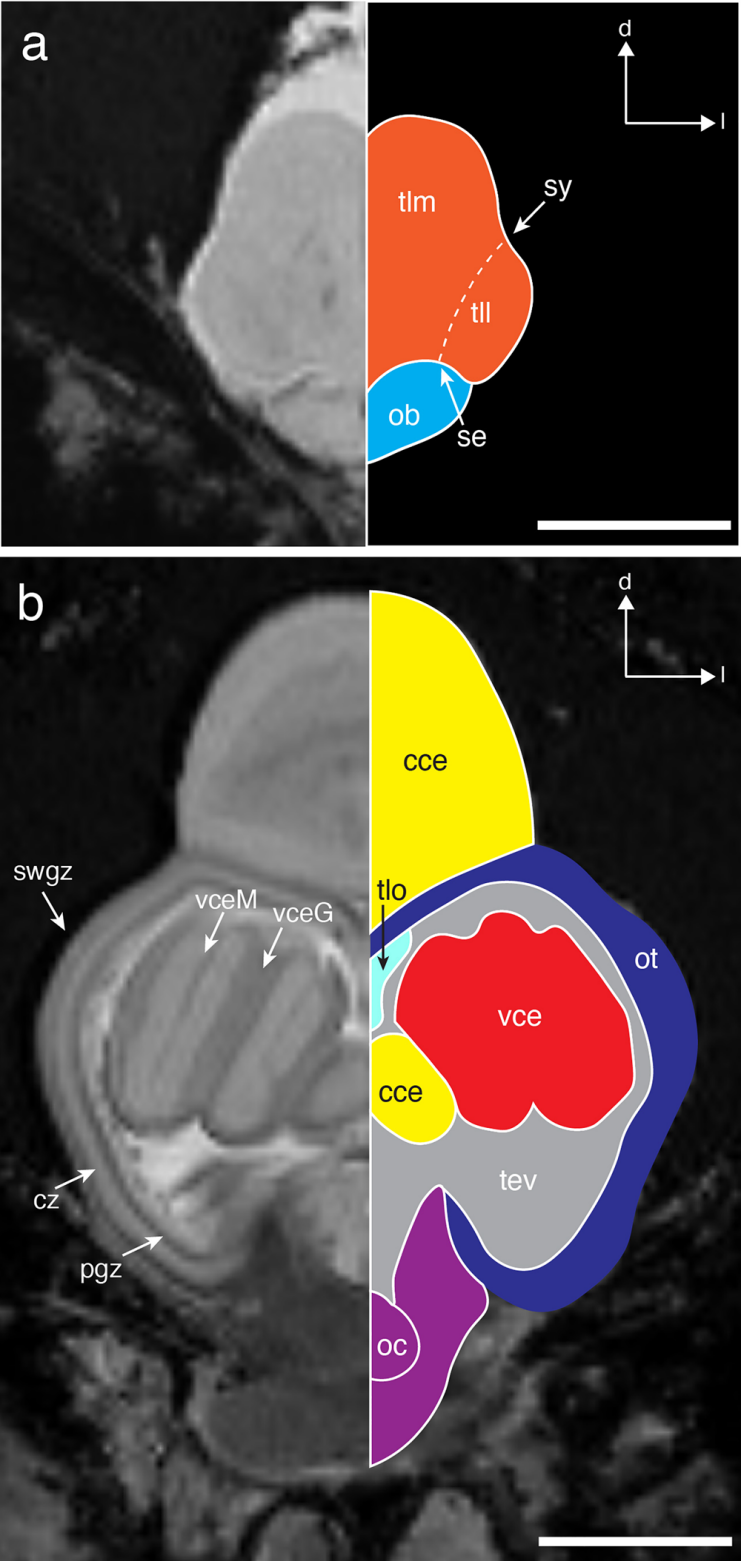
A pair of axial slices through the forebrain (a) and midbrain (b) of *T. maccoyii*. MRI 2D slices are on the left, and the line tracings on the right indicate different regions. Each region is colored with colors corresponding to the 3D segmentations, while the ventricle and areas that cannot be differentiated are rendered in gray). Dashed line indicates a likely boundary. Note that the optic tectum only comprises the roof of the midbrain. Orientation: d, dorsal; l, lateral. cc, corpus cerebelli; cs, connective sheath; cz, central zone of the ot; ob, olfactory bulb; oc, optic chiasma; ot, optic tectum; pgz, periventricular gray zone of the ot; se, sulcus externus; swgz, superficial gray and white zone of the ot; sy, sulcus ipsilyformis; tev, tectal ventricle; tll, telencephalon lateral lobe; tlm, telencephalon medial lobe; tlo, longitudinal torus; vce, valvula of cerebelli; vceG, granular layer of the vce; vceM, molecular layer of the vce. Scale bars, 5 mm (a, b).
